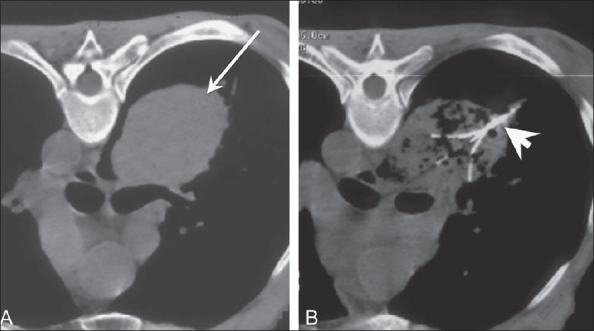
Bronchogenic carcinoma. Pre-ablation axial CT scan in the prone position (A) shows a large mass (arrow) in the lung. A similar image obtained during ablation (B) shows the electrode (arrowhead) in the mass
